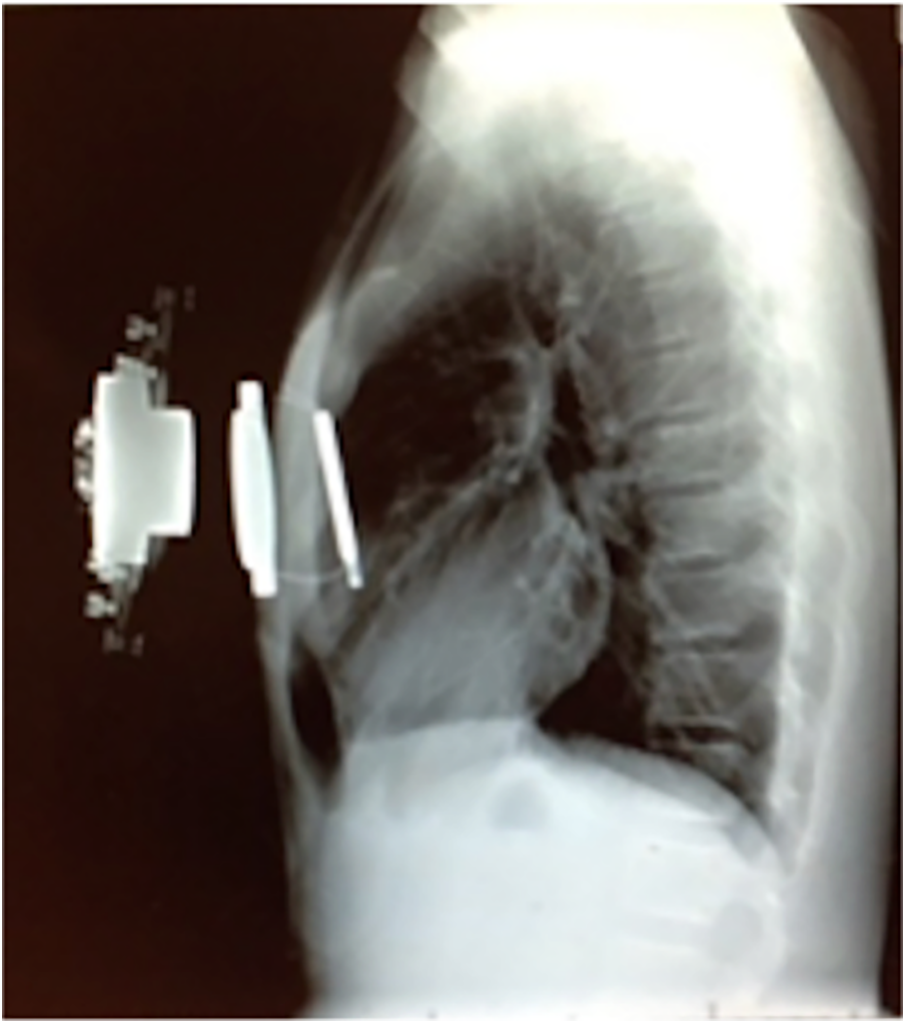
Lateral chest radiograph of a patient with pectus excavatum implanted with the magnimplant device and wearing the magnatract external brace. (Permission for use granted by Magnets-in-Me Inc., San Francisco, CA, USA).
